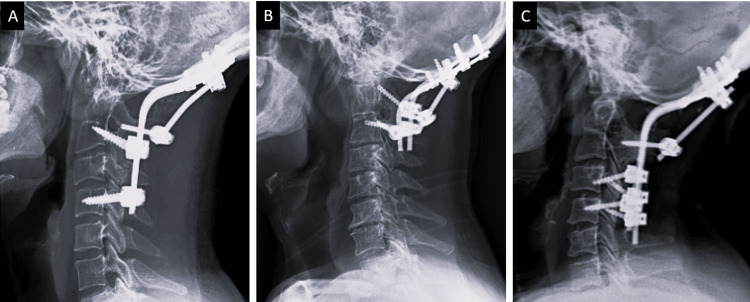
Representative follow-up lateral radiographs of patients operated for OC fusion with multiple rod construct. (A) Lateral radiograph of cervical spine at 12 months follow-up, who underwent occiput-C5 fusion with a three-rod construct. (B) Another patient's lateral radiograph of cervical spine at 14 months follow-up who underwent O-C3 fusion. (C) Seventeen months follow-up lateral radiograph of another patient operated on with O-C5 fusion. All the patients exhibit fusion and improvement in radiological parameters. OC fusion = Occipito-cervical fusion
